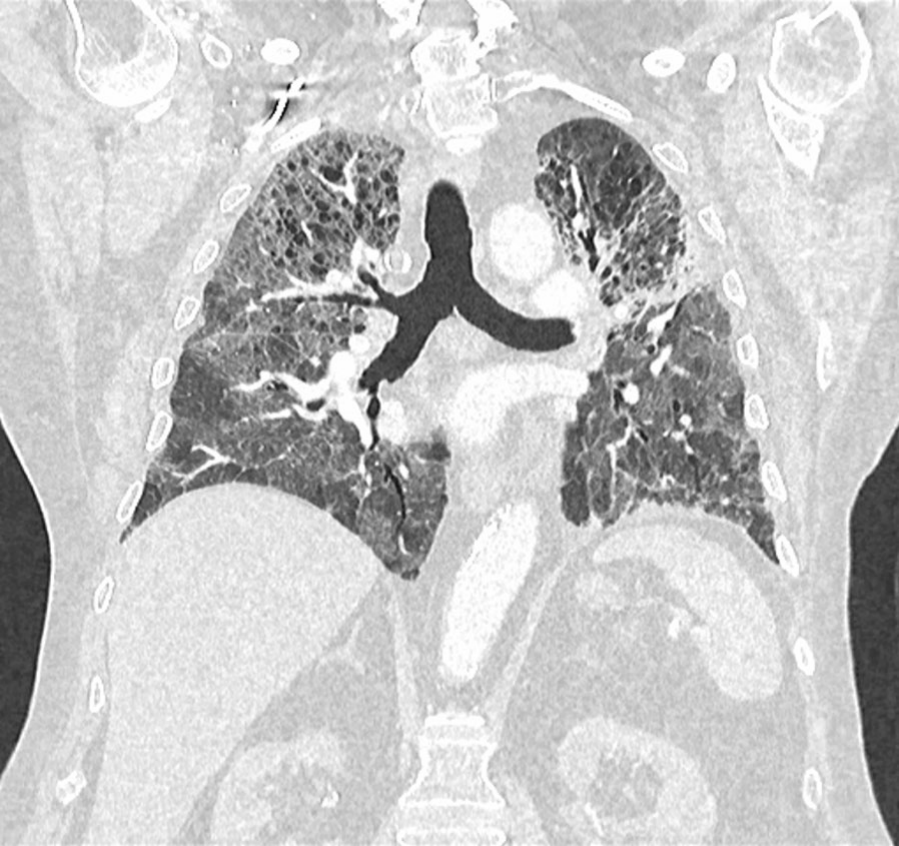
Case 3—CT chest after relapse of pneumonitis. There is no change in size of lung cancer
